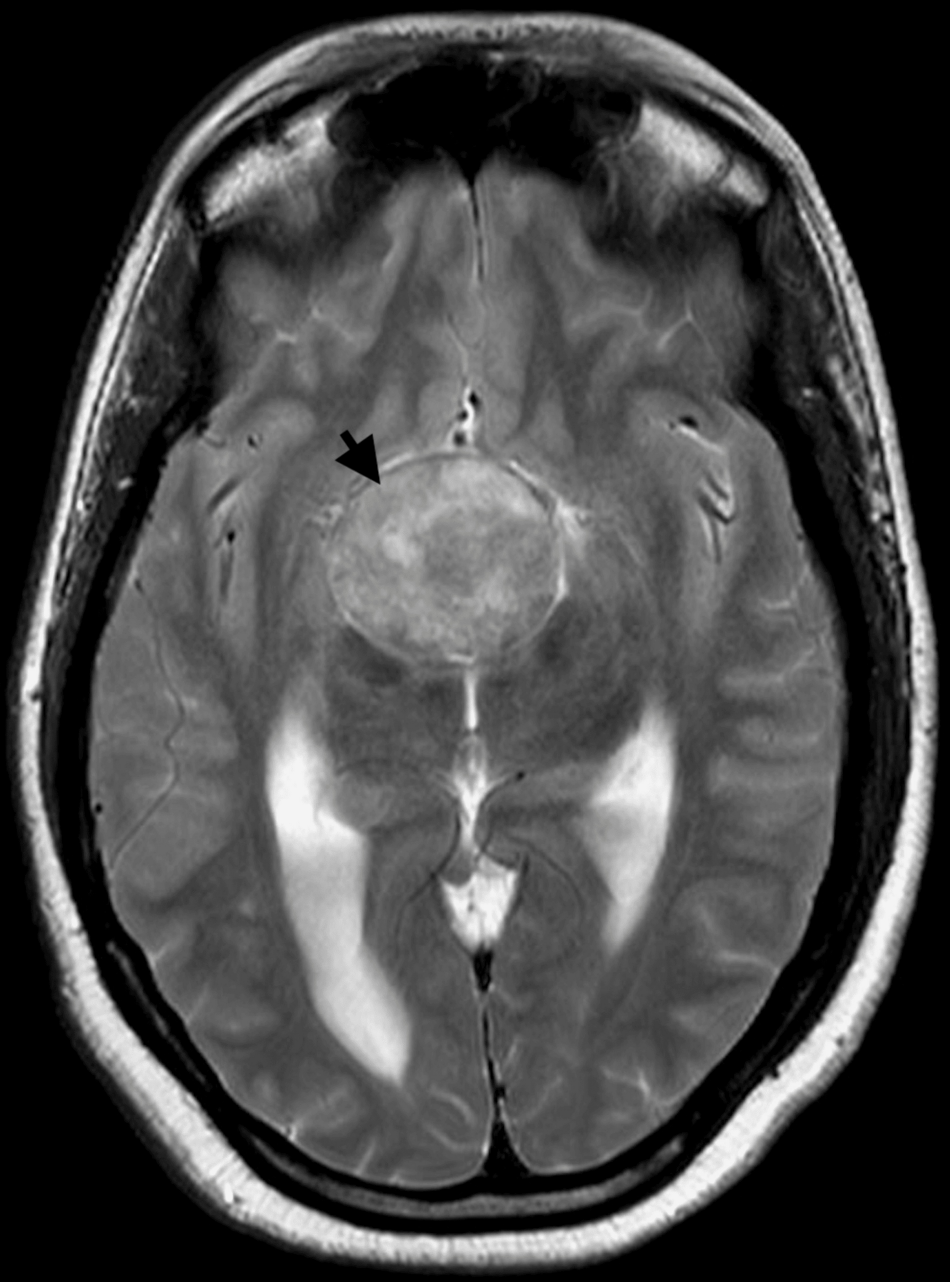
T2-weighted transverse sections: The lesion (black arrow) abuts bilateral internal carotid arteries (Knosp classification of cavernous sinus invasion by pituitary macroadenomas—Grade 2 on the right and grade 3A on the left side). It is reaching superiorly up to the floor of the third ventricle, compressing it, with consequent mild dilatation of bilateral lateral ventricles with periventricular hyperintensities. Posteriorly, it is splaying cerebral peduncles. Anteriorly indenting the fornix and bilateral anterior carotid arteries, which are draped over it. The lesion causes superior displacement of the optic nerves and complete effacement of the optic chiasma. Pituitary macroadenoma marked with a black arrowhead
